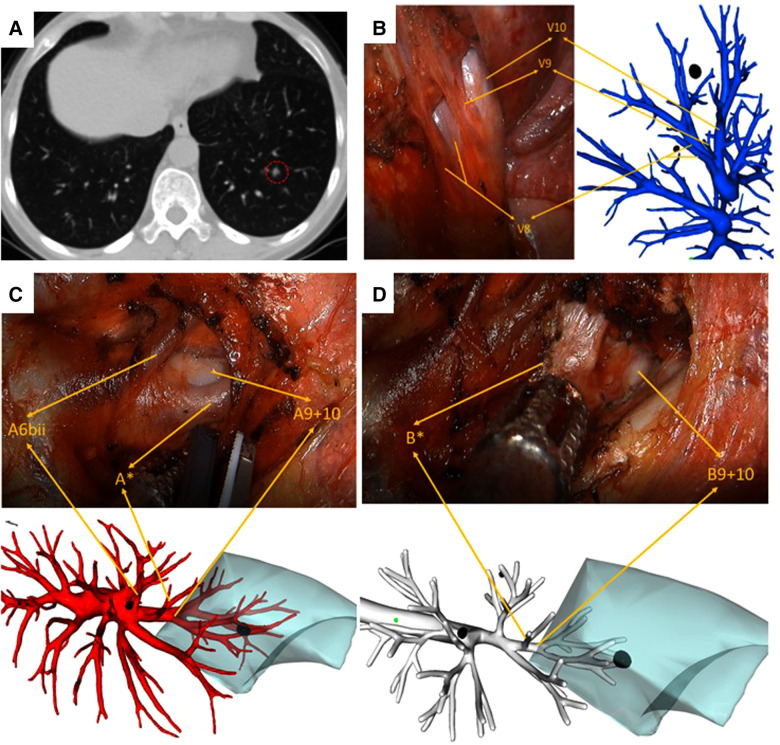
Left S9 VATS segmentectomy under real-time 3D reconstruction guidance (with the subsuperior segment S*). (A) preoperative CT scan showing a 6 mm GGN lesion in the left S9. (B) Branches of the pulmonary vein, (C) branches of the pulmonary artery, including A*, and (D) branches of the bronchus, including B*, compared by using the real-time guidance of 3D reconstruction.
